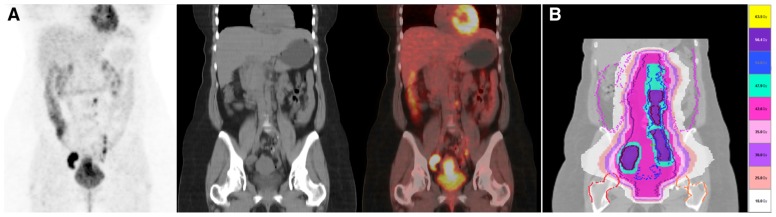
(A) A 48 year-old lady with a cervical cancer stage FIGO IIB, presenting with multiple positive lymph nodes in continuity located in the bilateral iliac and para-aortic regions on FDG PET/CT. She was treated with chemo-radiotherapy using helical TomoTherapy. (B) Three level of radiotherapy dose were design and treated simultaneously. Pelvis and para-aortic areas received 44.8 Gy/1.6 Gy in 28 fractions. The PAO and pelvis regions surrounding positive nodes but without metabolic uptake were treated with 50.4 Gy/1.8 Gy in 28 fractions. Positive FDG PET/CT lymph nodes were treated with a simultaneous integrated boost up to 59.36 Gy/2.12 Gy in 28 fractions. Scale dose banding shows the 95% of the dose.
